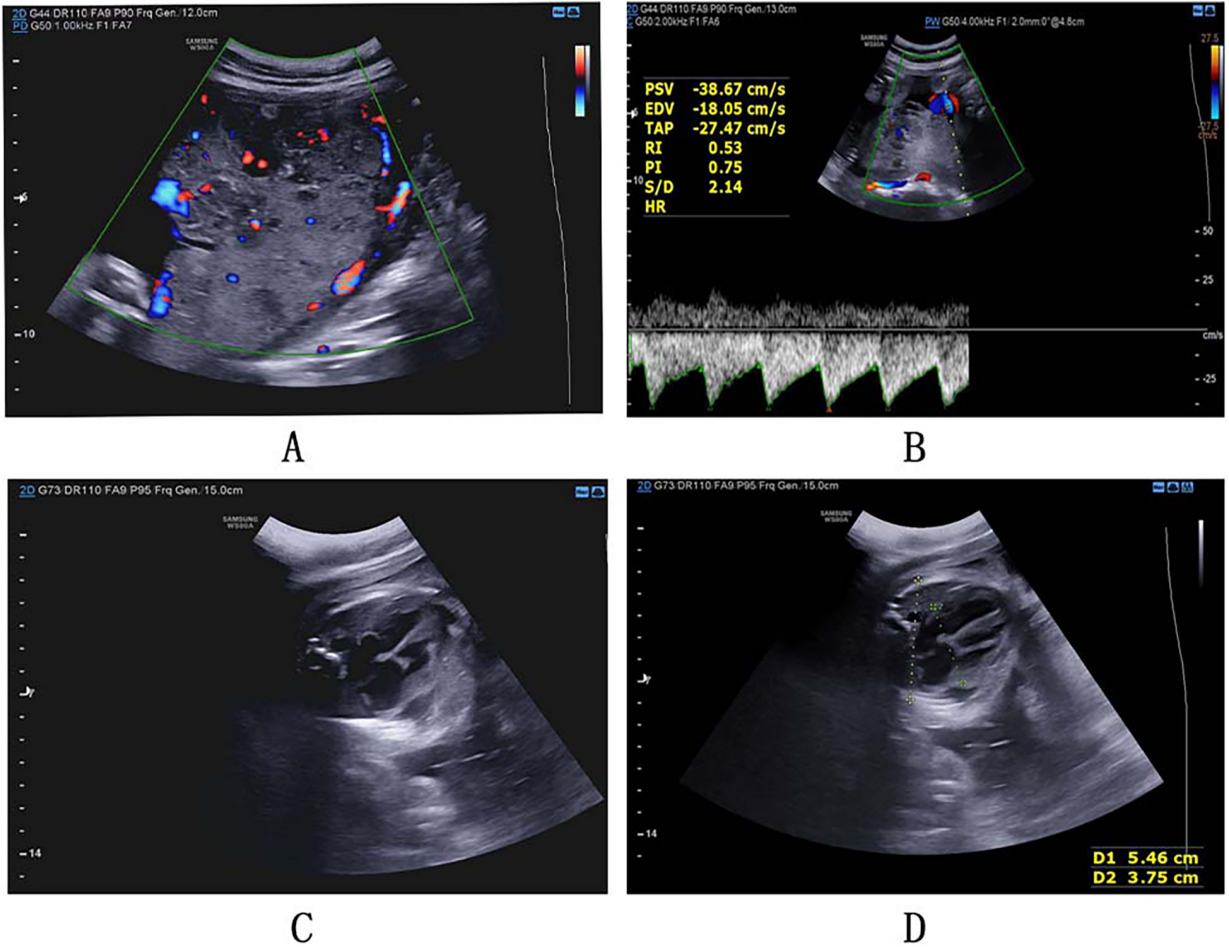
(A) The placenta was thickened (placenta thickness 5.7 cm), with multiple irregular low-weak echoes in the parenchyma, some of which showed honeycomb changes; (B) the fetal color Doppler showed that PSV was greater than 1.5 MOM; (C) the fetal heart grew up with pericardial effusion; (D) increased fetal cardiothoracic ratio.
